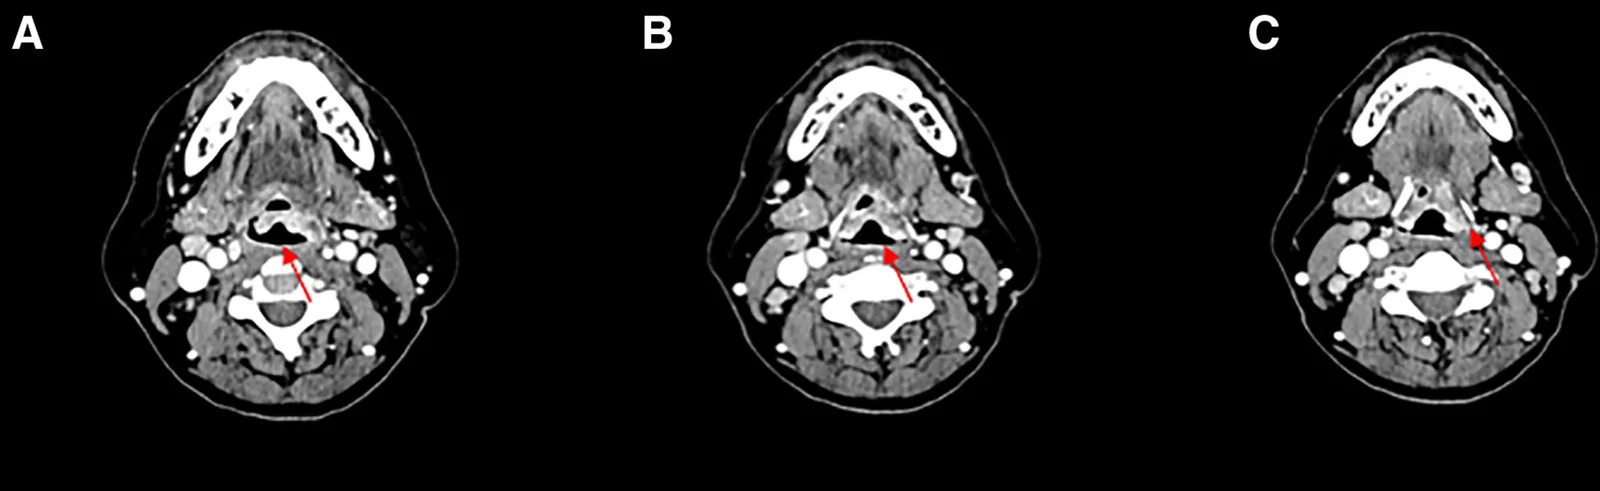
Computed tomography imaging of case 1. (A–C) Computed tomography images of 3 different levels of the pyriform fossa. Thickening and enhancement of the left epiglottic fold, with concomitant narrowing of the left anterior epiglottic space and the pyriform fossa.
